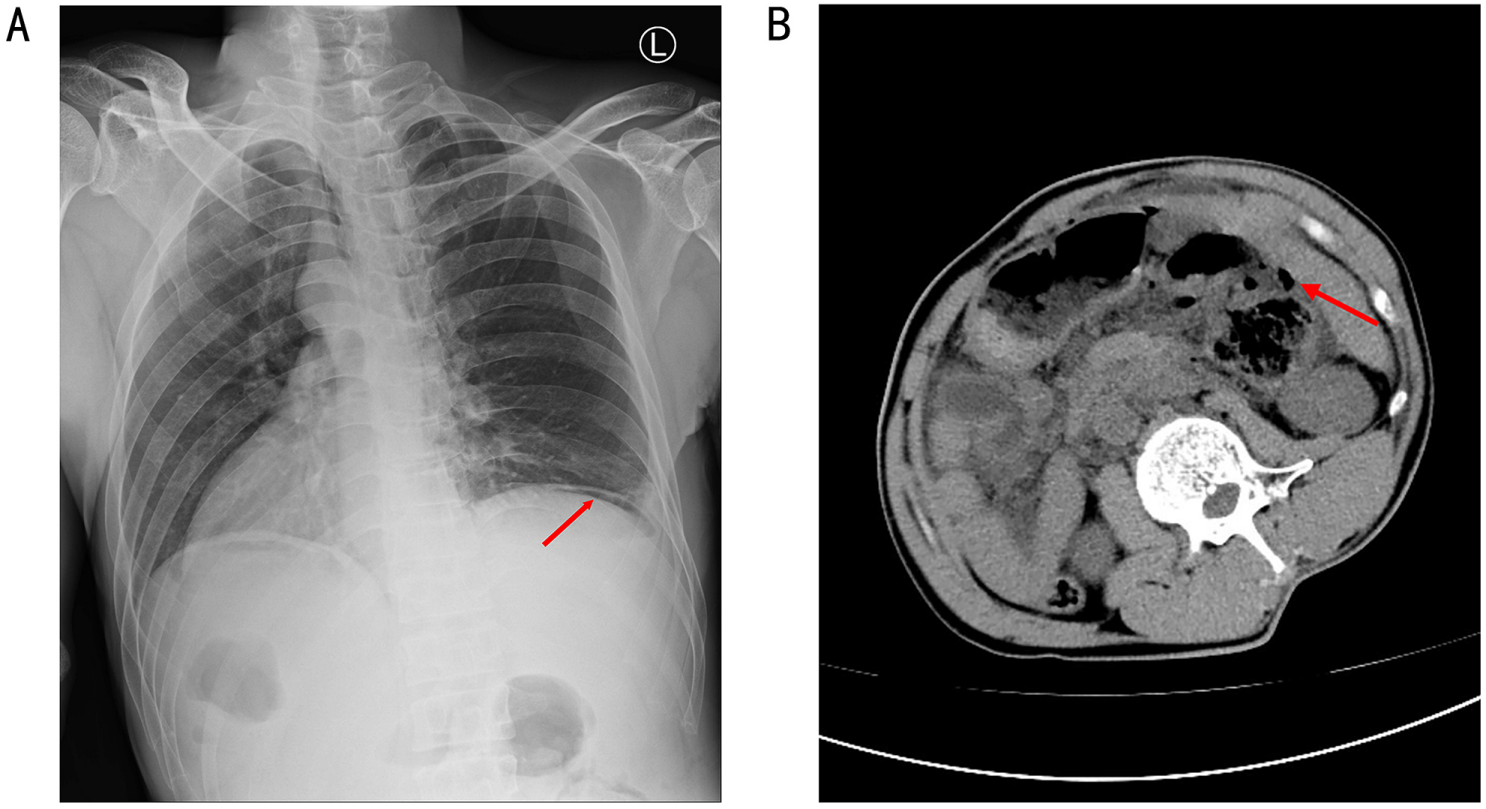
A chest and abdominal CT revealed free gas in the abdominal cavity, indicating a gastrointestinal perforation (A, B).
